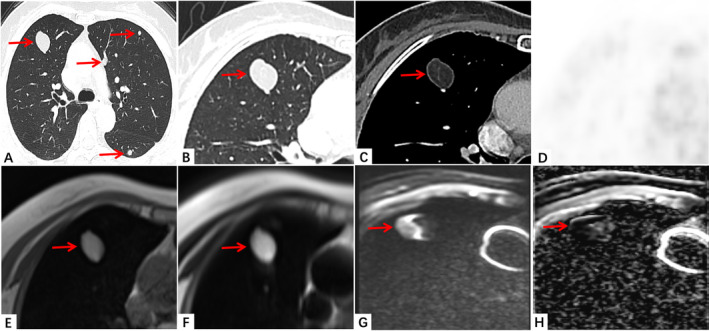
(A–H) The patient had CT MRI and PET‐CT examination. (A) Multiple metastatic nodules in both lungs. (B, C) The large nodular foci in the upper lobe of the right lung. CT contrast focus scan of nodules shows fat and vascular. The mean CT Value of this nodule was −91 HU. (D) No obvious uptake in PET‐CT at the same site. (E) The nodules are T1 high signal. (F) T2 high signal in MRI. (G) DWI high signal. (H) ADC low signal, diffusion is limited. The red arrows represented the lung nodules.
